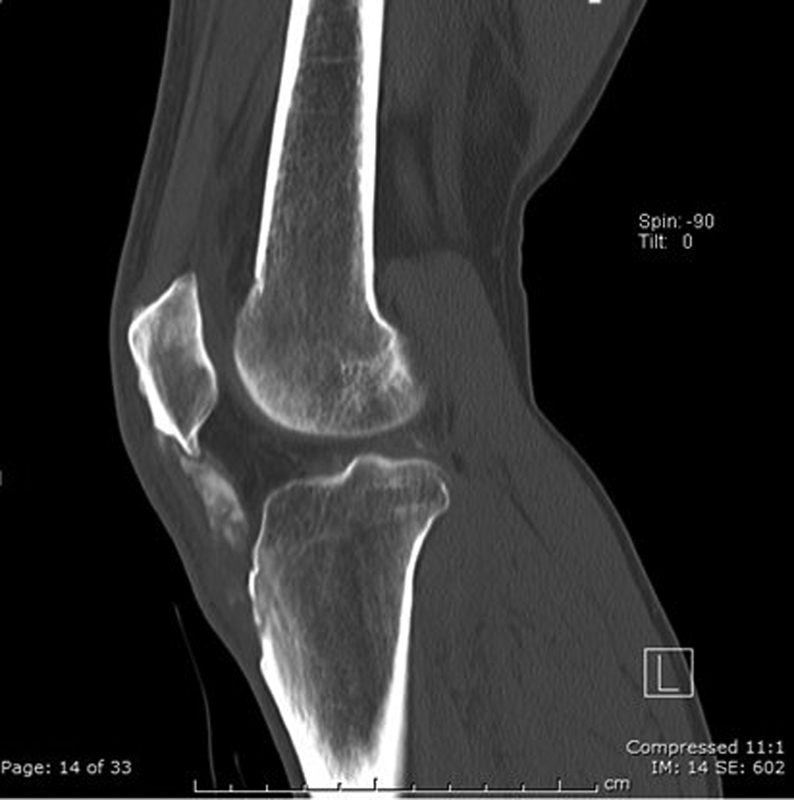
Computed tomography sagittal plane image.
